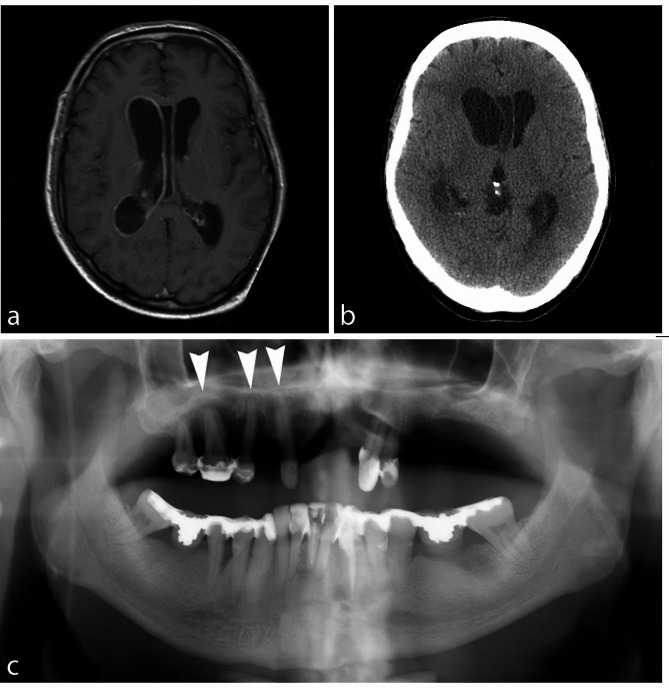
a. Gadolinium enhanced T1 MR image. The ependyma of the right lateral ventricle and the cavum septi pellucidi enhances intensively, as a sign of ventriculitis. Lateral ventricles are slightly enlarged. b. Unenhanced CT slice on the level of frontal horns. Hydrocephalus has progressed, especially on the right side, the right frontal horn has enlarged and the anterior part of cavum septi pellucidi deviates to the left. Cortical sulci are narrowed compared to the previous examination. c. General chronic periodontitis in both jaws and severe local bone loss with abscesses. Periapical abscess is indicated by arrow
